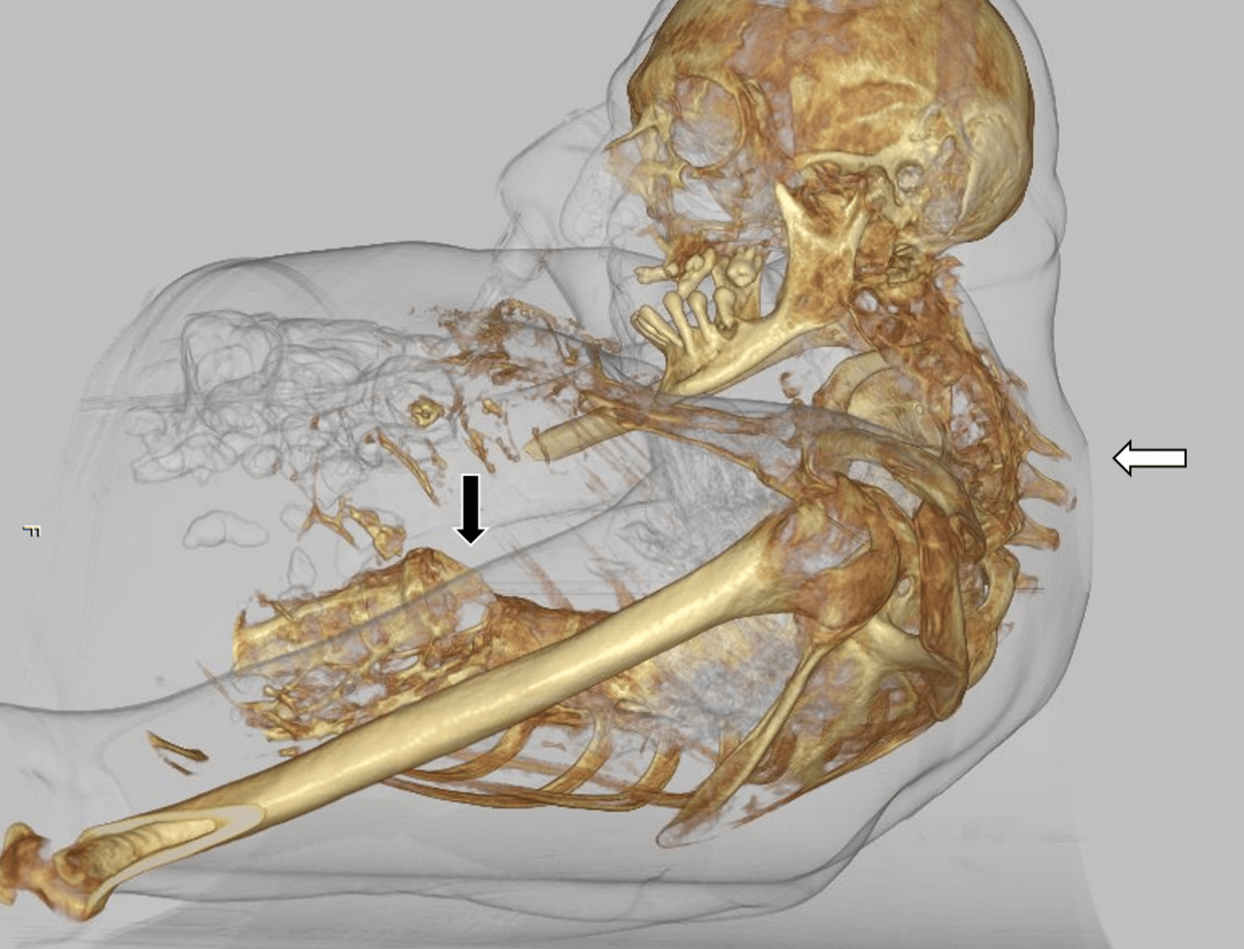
A 3D reconstruction of a CT scan revealing pronounced cervical deformities, characterized by severe hyperkyphosis and ankylosis (indicated by the white arrow), along with a spinal fracture (denoted by the black arrow). CT - computerized tomography
